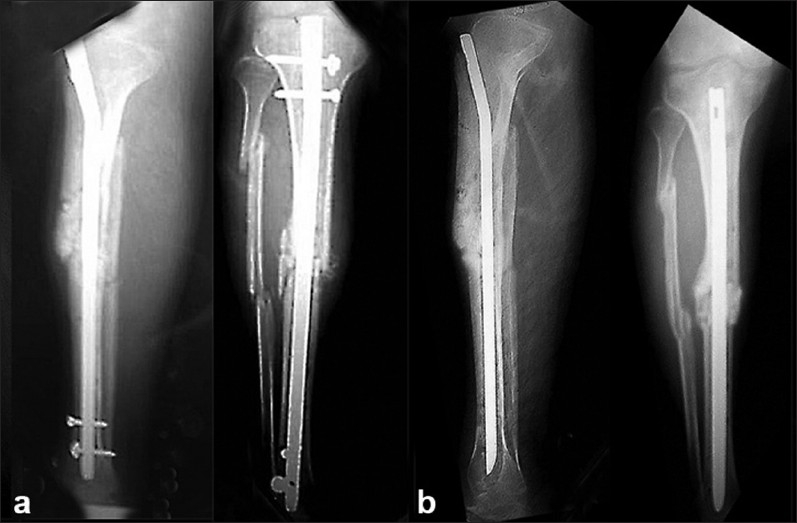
Lateral and anteroposterior (a) X-ray of a closed fracture middle 1/3rd tibia treated with conventional interlocking nail, which subsequently developed infection. Union was achieved with debridement and antibiotic cement-impregnated intramedullary nail and a radiograph taken at 18 months follow-up (b)
